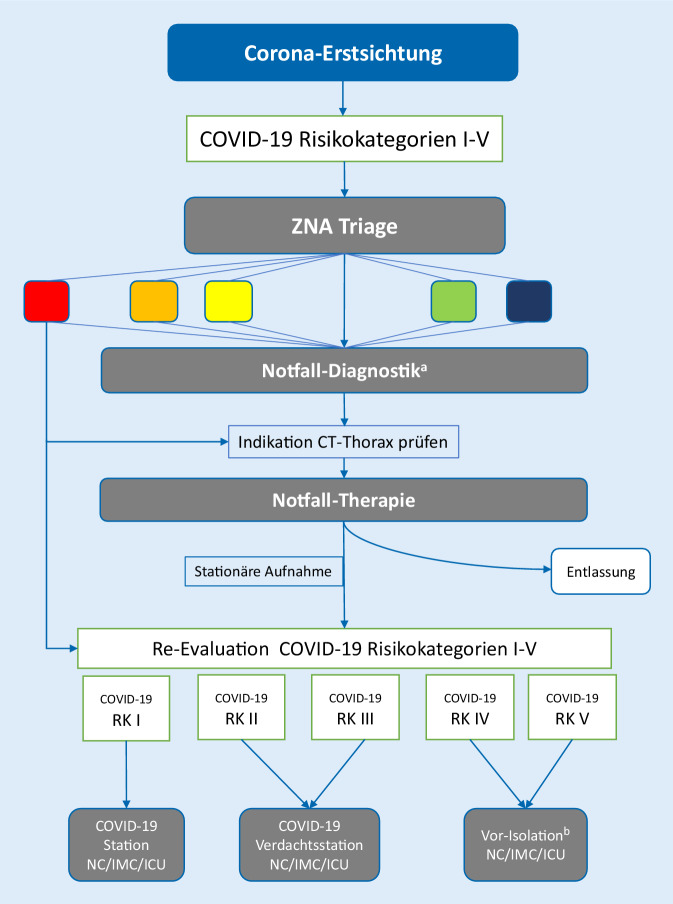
Zur Notfalldiagnostik bei COVID-19-Risikostatus II–IV wurde ein standardisiertes Vorgehen implementiert: Neben der Bestimmung der Vitalparameter (RR, Puls, S_p_O_2_) erfolgt eine Labordiagnostik (Blutbild, Differenzialblutbild, Kreatinin, Elektrolyte, LDH, PCT, CRP, aBGA). Bei allen Patienten erfolgt ein Nasen-Rachen-Abstrich und eine Asservierung von Rachenspülwasser für die PCR-Diagnostik auf SARS-CoV‑2 und ggf. für die Durchführung eines Influenza-Schnelltests (nur im Zeitraum der Influenza-Epidemie). Zur bildgebenden Diagnostik wurde als Standard die Thorax-Sonographie als fester Bestandteil der Diagnostik basierend auf den Empfehlungen von Volpicelli et al. [6] eingeführt. Pleuraergüsse, Pneumothorax oder die Zeichen der kardialen Insuffizienz als Ursache der respiratorischen Insuffizienz sollen hierdurch primär erkannt und auf aufwändige Patiententransporte in die Röntgenabteilung verzichtet werden. Bei begründeten Verdachtsfällen (COVID-Risikokategorie II) oder bei hoher klinischer Wahrscheinlichkeit für COVID-19, Nachweis einer Lymphopenie und negativem Procalcitonin wird bei indizierter stationärer Behandlung eine Low-dose-CT-Untersuchung des Thorax durchgeführt [7–9] und gemäß CO-RADS-Kategorien [10] klassifiziert. Nach Durchführung der Notfalldiagnostik erfolgt bei stationär zu behandelnden Patienten eine Reevaluation der COVID-19-Risikostatus basierend auf den Untersuchungsergebnissen und nachfolgend die Auswahl der jeweiligen Zielstation gemäß Kategorisierung (Tab. 1).
